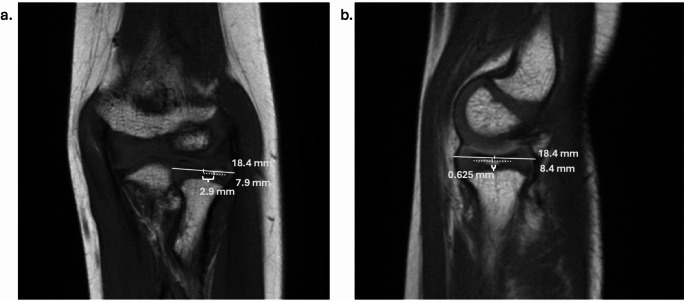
Exemplar measurements of the cartilaginous anlage in the coronal and sagittal planes. (a) Coronal view demonstrating measurements of the cartilaginous anlage (18.4 mm), radial head ossific nucleus (7.87 mm), and radial head offset of 3.93 mm radially. (b) Sagittal MRI image demonstrating measurements of the cartilaginous anlage (18.4 mm), radial head ossific nucleus (8.44 mm), and anterior (positive) radial head offset of 0.625 mm
